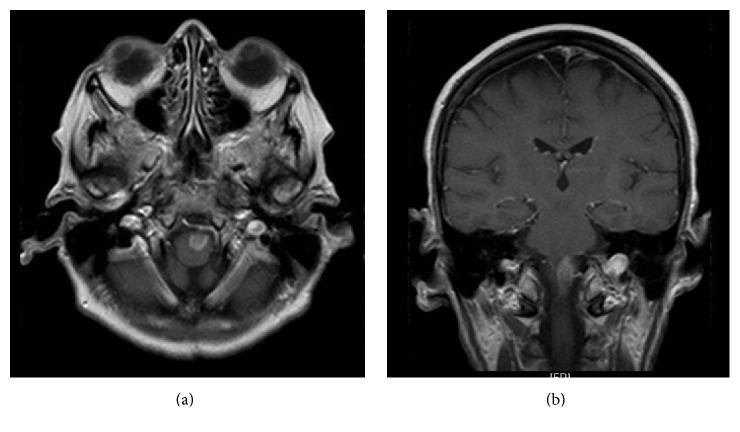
Initial brain MRI. (a) Axial T1-weighted image with gadolinium, showing the enhancing left medullary lesion. (b) Coronal T1-weighted image with gadolinium showing the same medullary lesion.
